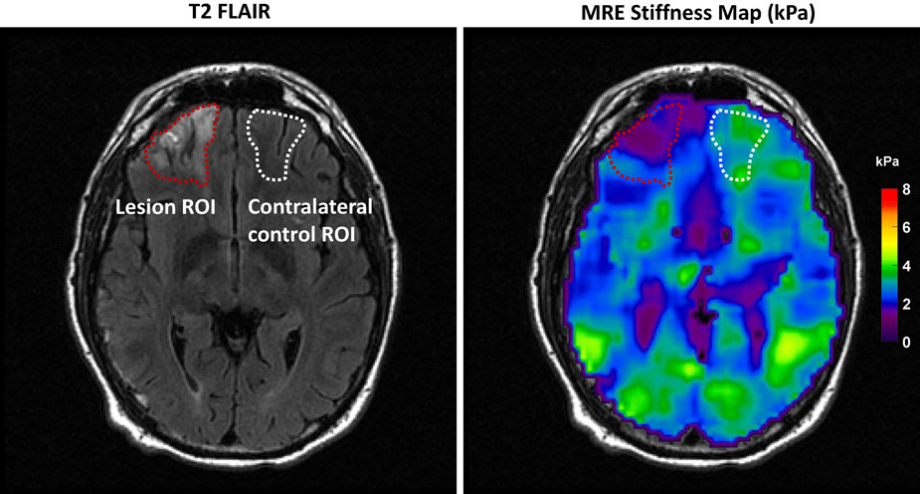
Examples of lesion region of interest and its contralateral control region of interest, delineated on the T2 fluid attenuated inversion recovery (FLAIR) image, and then registered and resampled to the magnetic resonance elastography (MRE) space.
